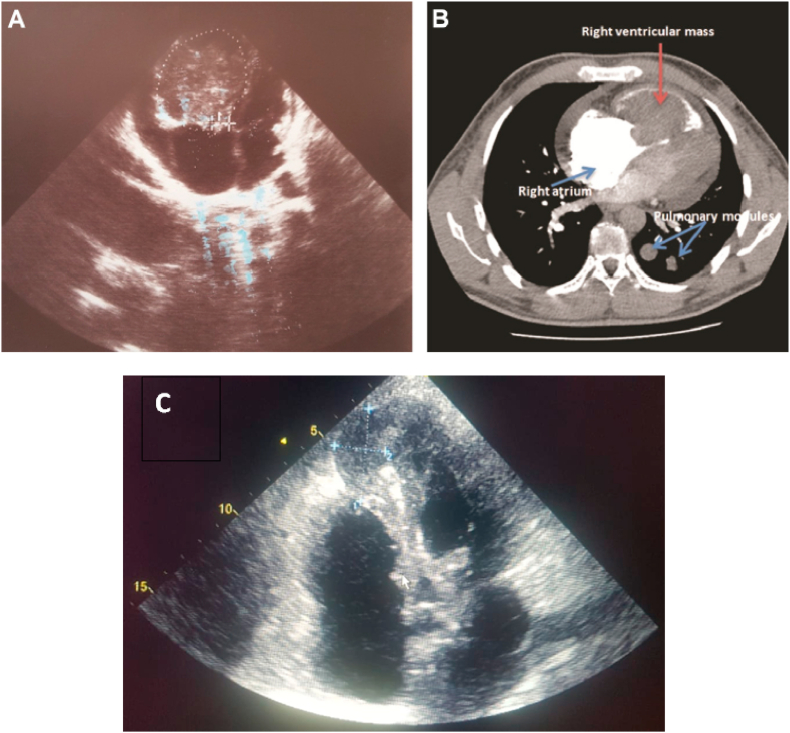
(A; B;C) Transthoracic echocardiography and Chest computed tomography imaging showing a right ventricular metastasis of a testicular non seminomatous germ cell tumor. (C) Transthoracic echocardiography imaging showing a right ventricular metastasis of a tumor infiltrated hilar and mediastinal lymph nodes.
